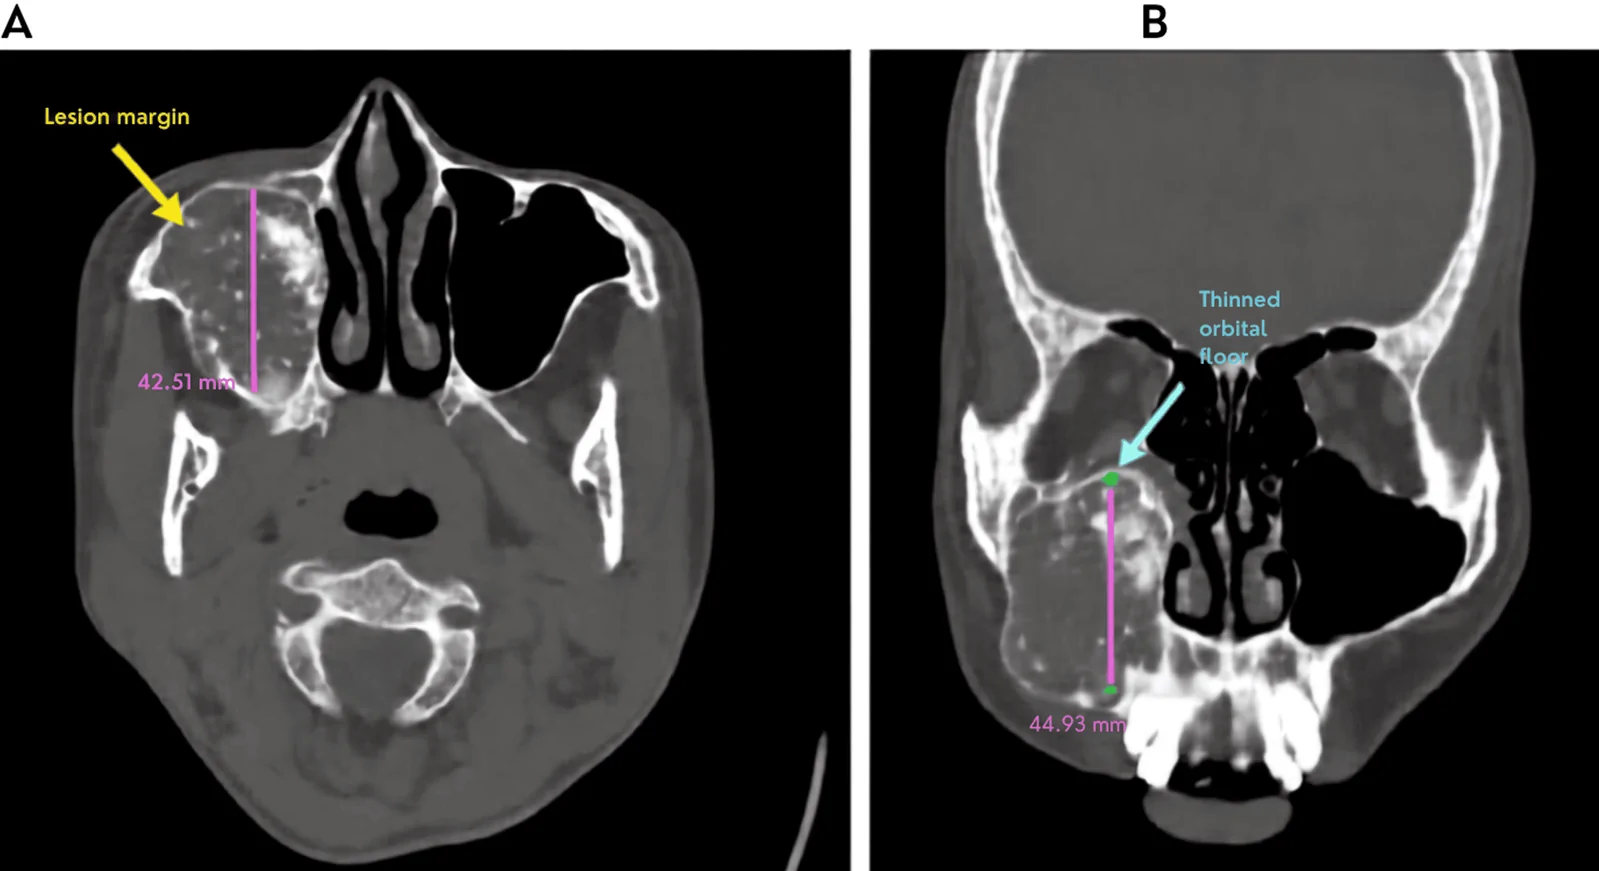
Preoperative computed tomography (A) Axial bone-window image showing the well-circumscribed margin of the mixed-density expansile lesion occupying the right maxillary sinus. (B) Coronal bone-window image demonstrating superior extension of the lesion and thinning of the right orbital floor, which remained radiologically intact.
